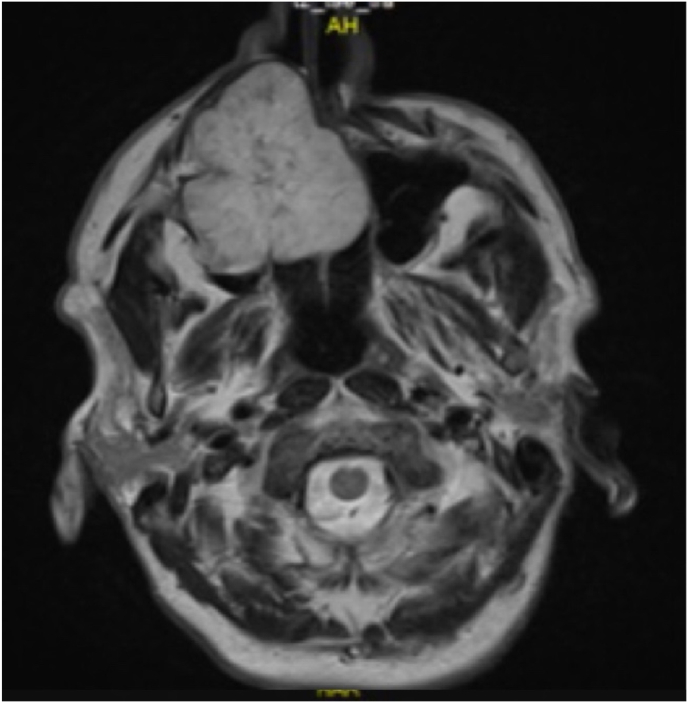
Brain MRI showed right maxillary sinus mass extending to the nasal cavity and two small osteolytic skull lesions.
